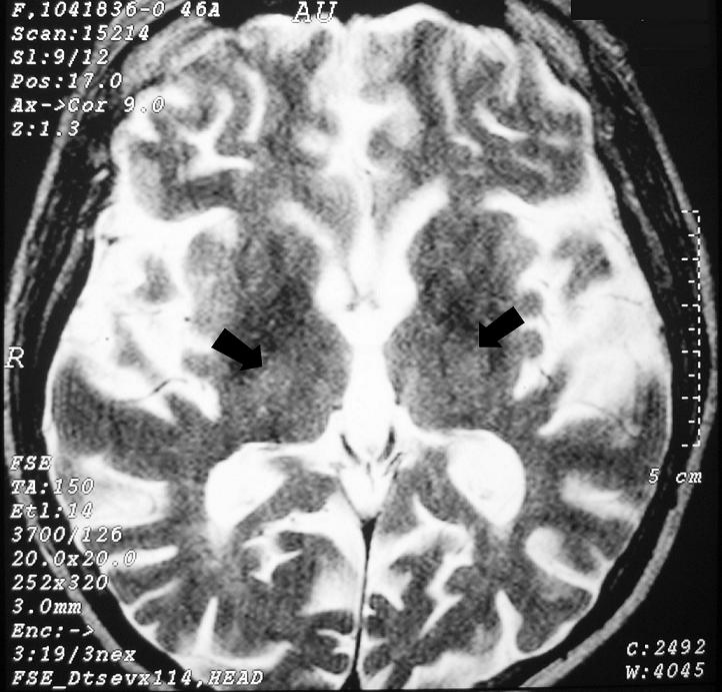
Brain axial T2W magnetic resonance imaging at the level of the thalami and third ventricle. High signal foci in the medial regions of thalami (arrows).
